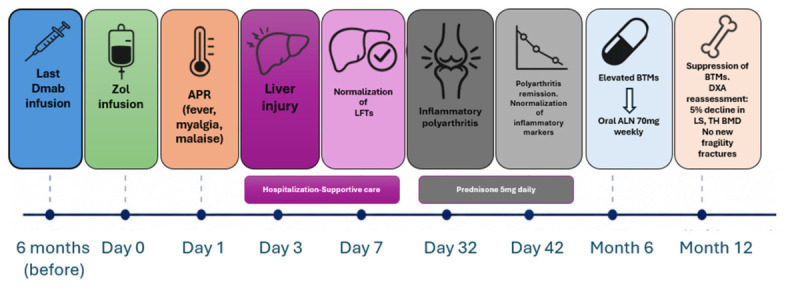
Chronological sequence of adverse events, treatment interventions, and skeletal outcomes following ZOL infusion. Abbreviations: Dmab, denosumab; ZOL, zoledronate; APR, acute phase response; LFTs, liver function tests; BTMs, bone turnover markers; ALN, alendronate; LS, lumbar spine; TH, total hip; BMD, bone mineral density, DXA, dual-energy X-ray absorptiometry. Different colors represent different categories of clinical events. Dashed vertical lines indicate the timing of each event along the study timeline.
